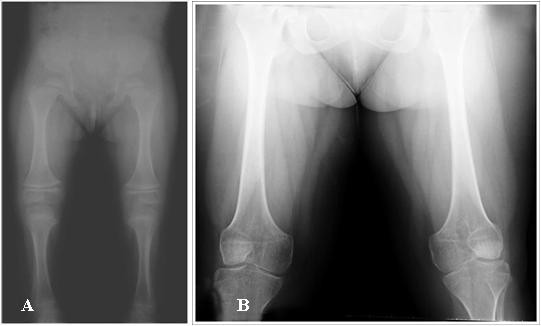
Radiographs of patient 1. A. 4 years. B. 28 years. Note short iliac bones, flattened femoral heads, short and broad femoral neck. Short femoral and tibial diaphysys, methaphyseal widening and broad femoral ends.
